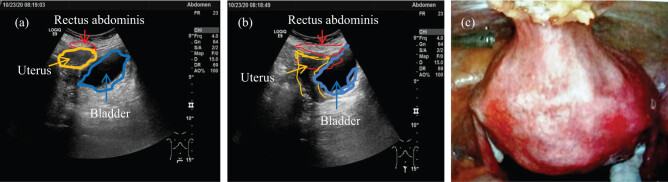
Ultrasound evaluation of postoperative effects of LRAHUP and clinical evidence of permanent adhesion.
